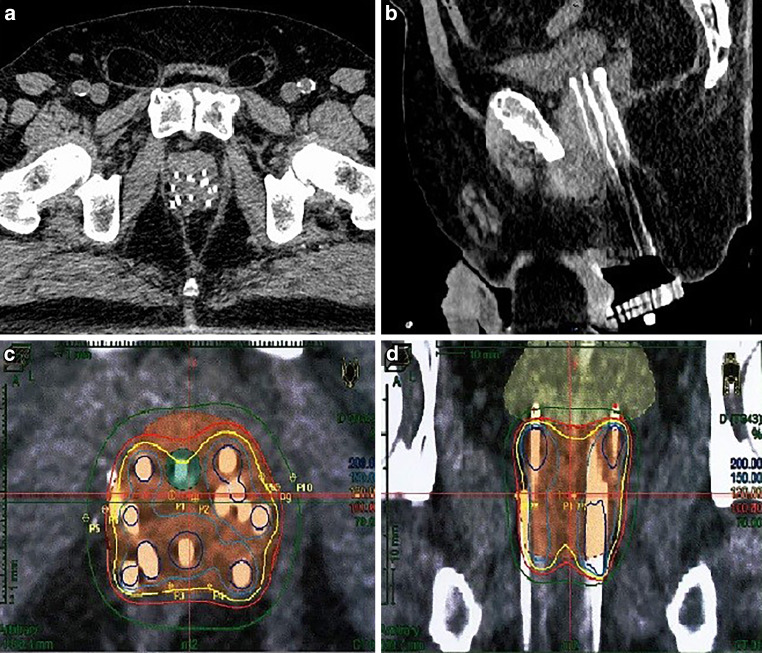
Example of CT-guided salvage brachytherapy in a patient after rectal extirpation; a axial view, b sagittal view, c, d corresponding dose distribution
